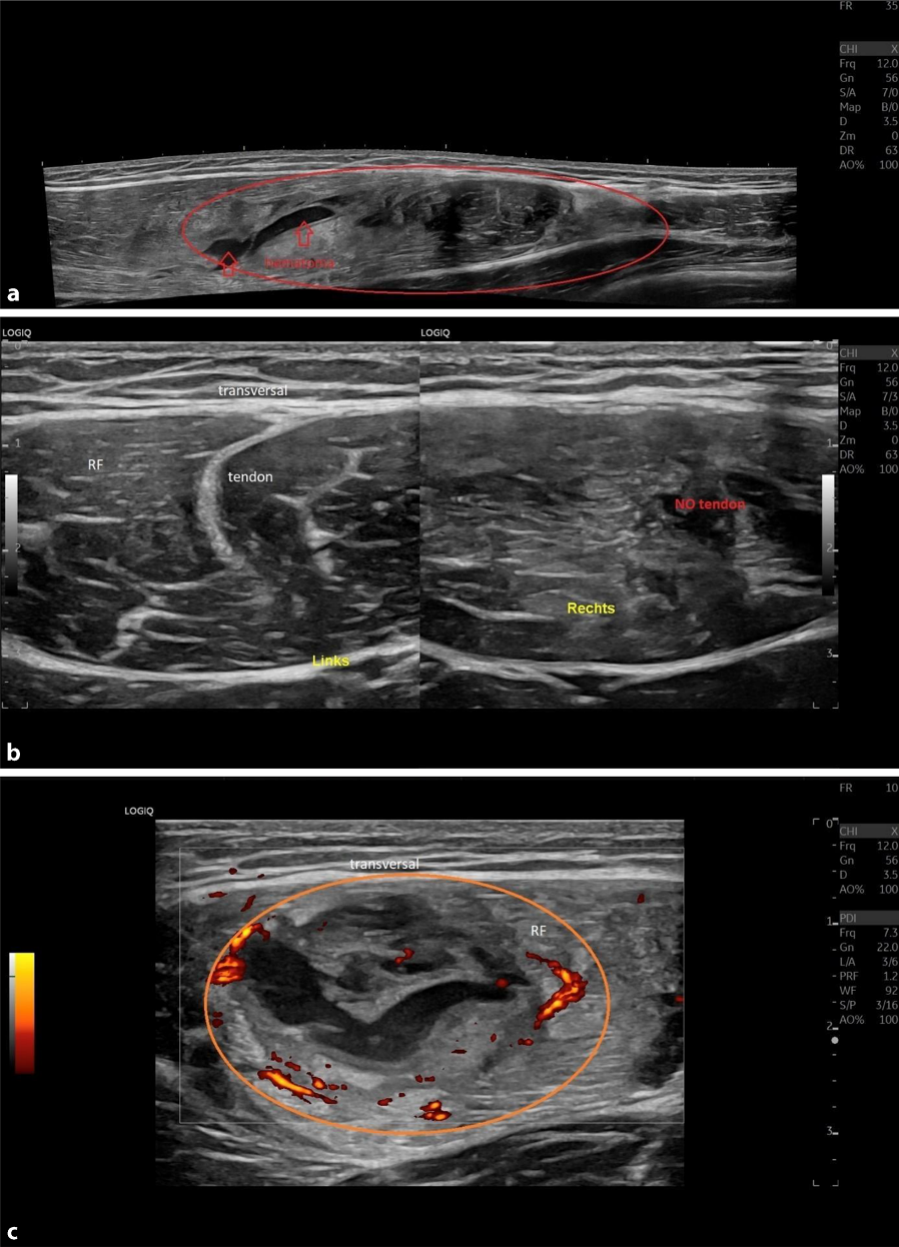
## Doppler-Ultraschall, z. B. Farb-Doppler (C-Doppler), Power-Doppler, B-Flow, MVI
